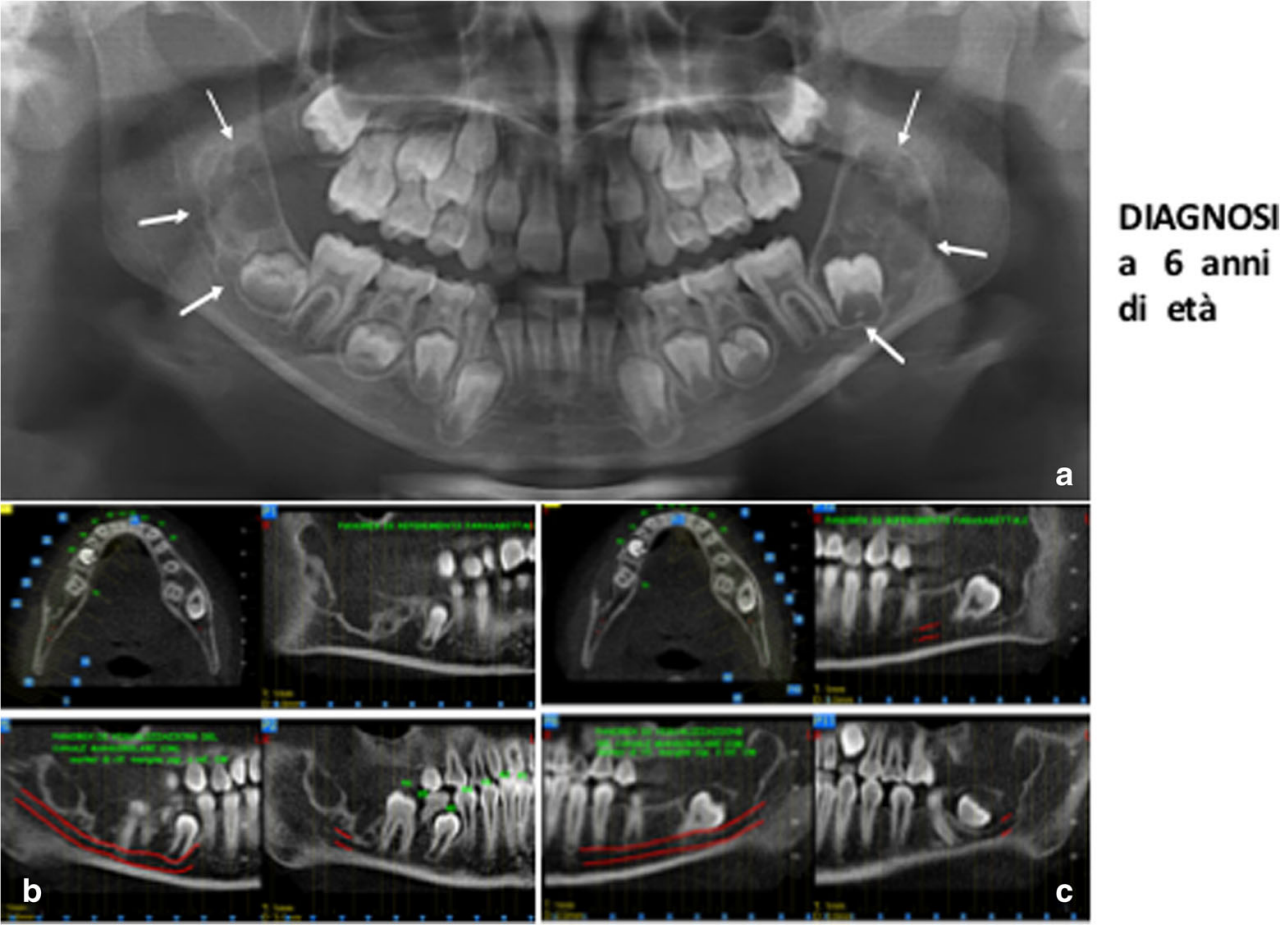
TC, female, diagnosis at age 6 (29-mar-2012) - a Panoramic radiography shows on both side of the mandible two symmetric large multilocular radiolucent lesions involving the angle and the ramus regions and the second molars. (white arrows). In the lower dental arch there are the first and the second molars (b, c) CTCB study of the mandible, respectively, of the right and the left site, shows the extension of the lesions. Note their critical relationship with the mandibular canal and its neurovascular structures, in particular the inferior alveolar nerves
